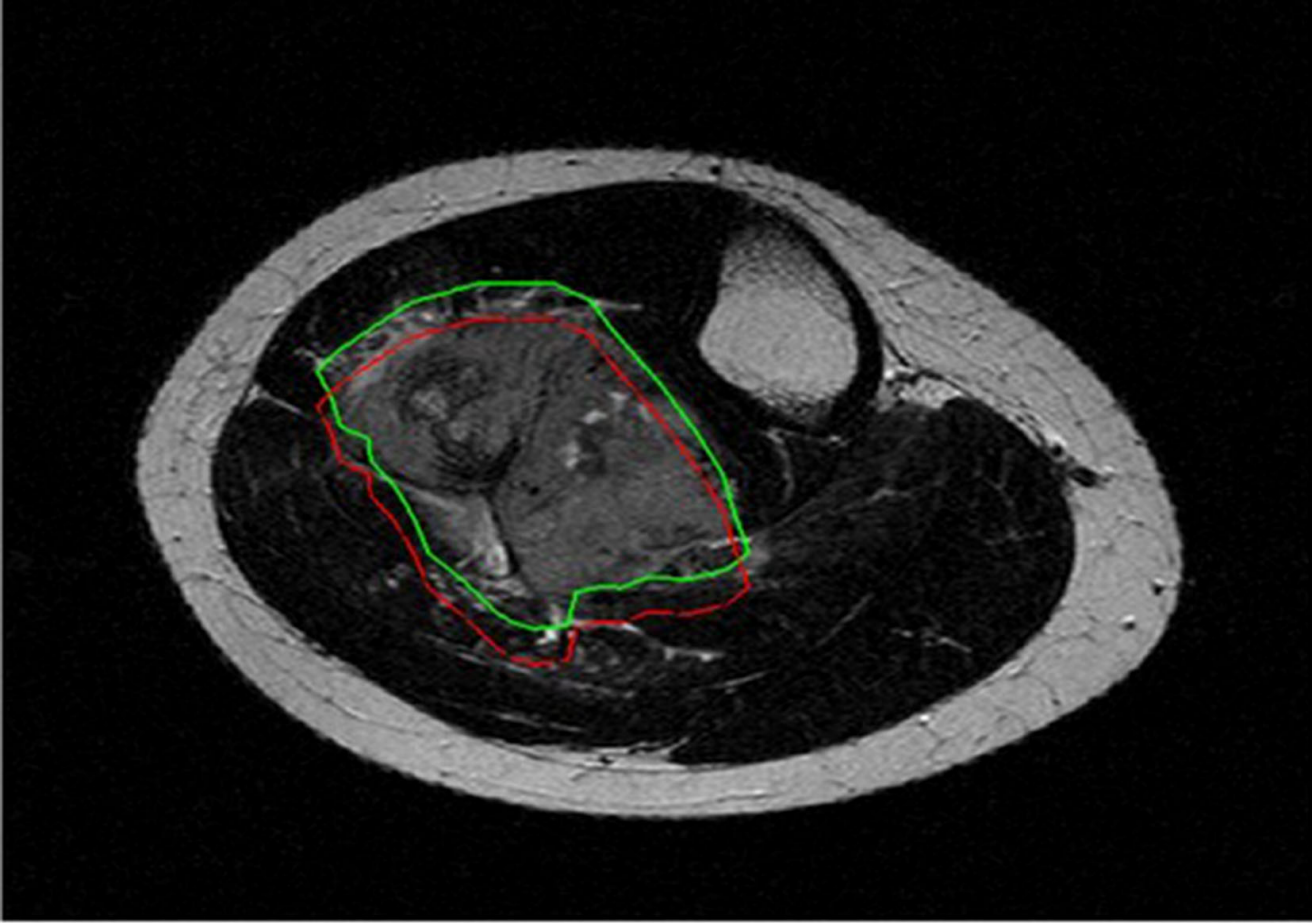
Original (green) and translated (red) version of 2D region of interest drawn around tumor borders on T2-weighted axial image.
